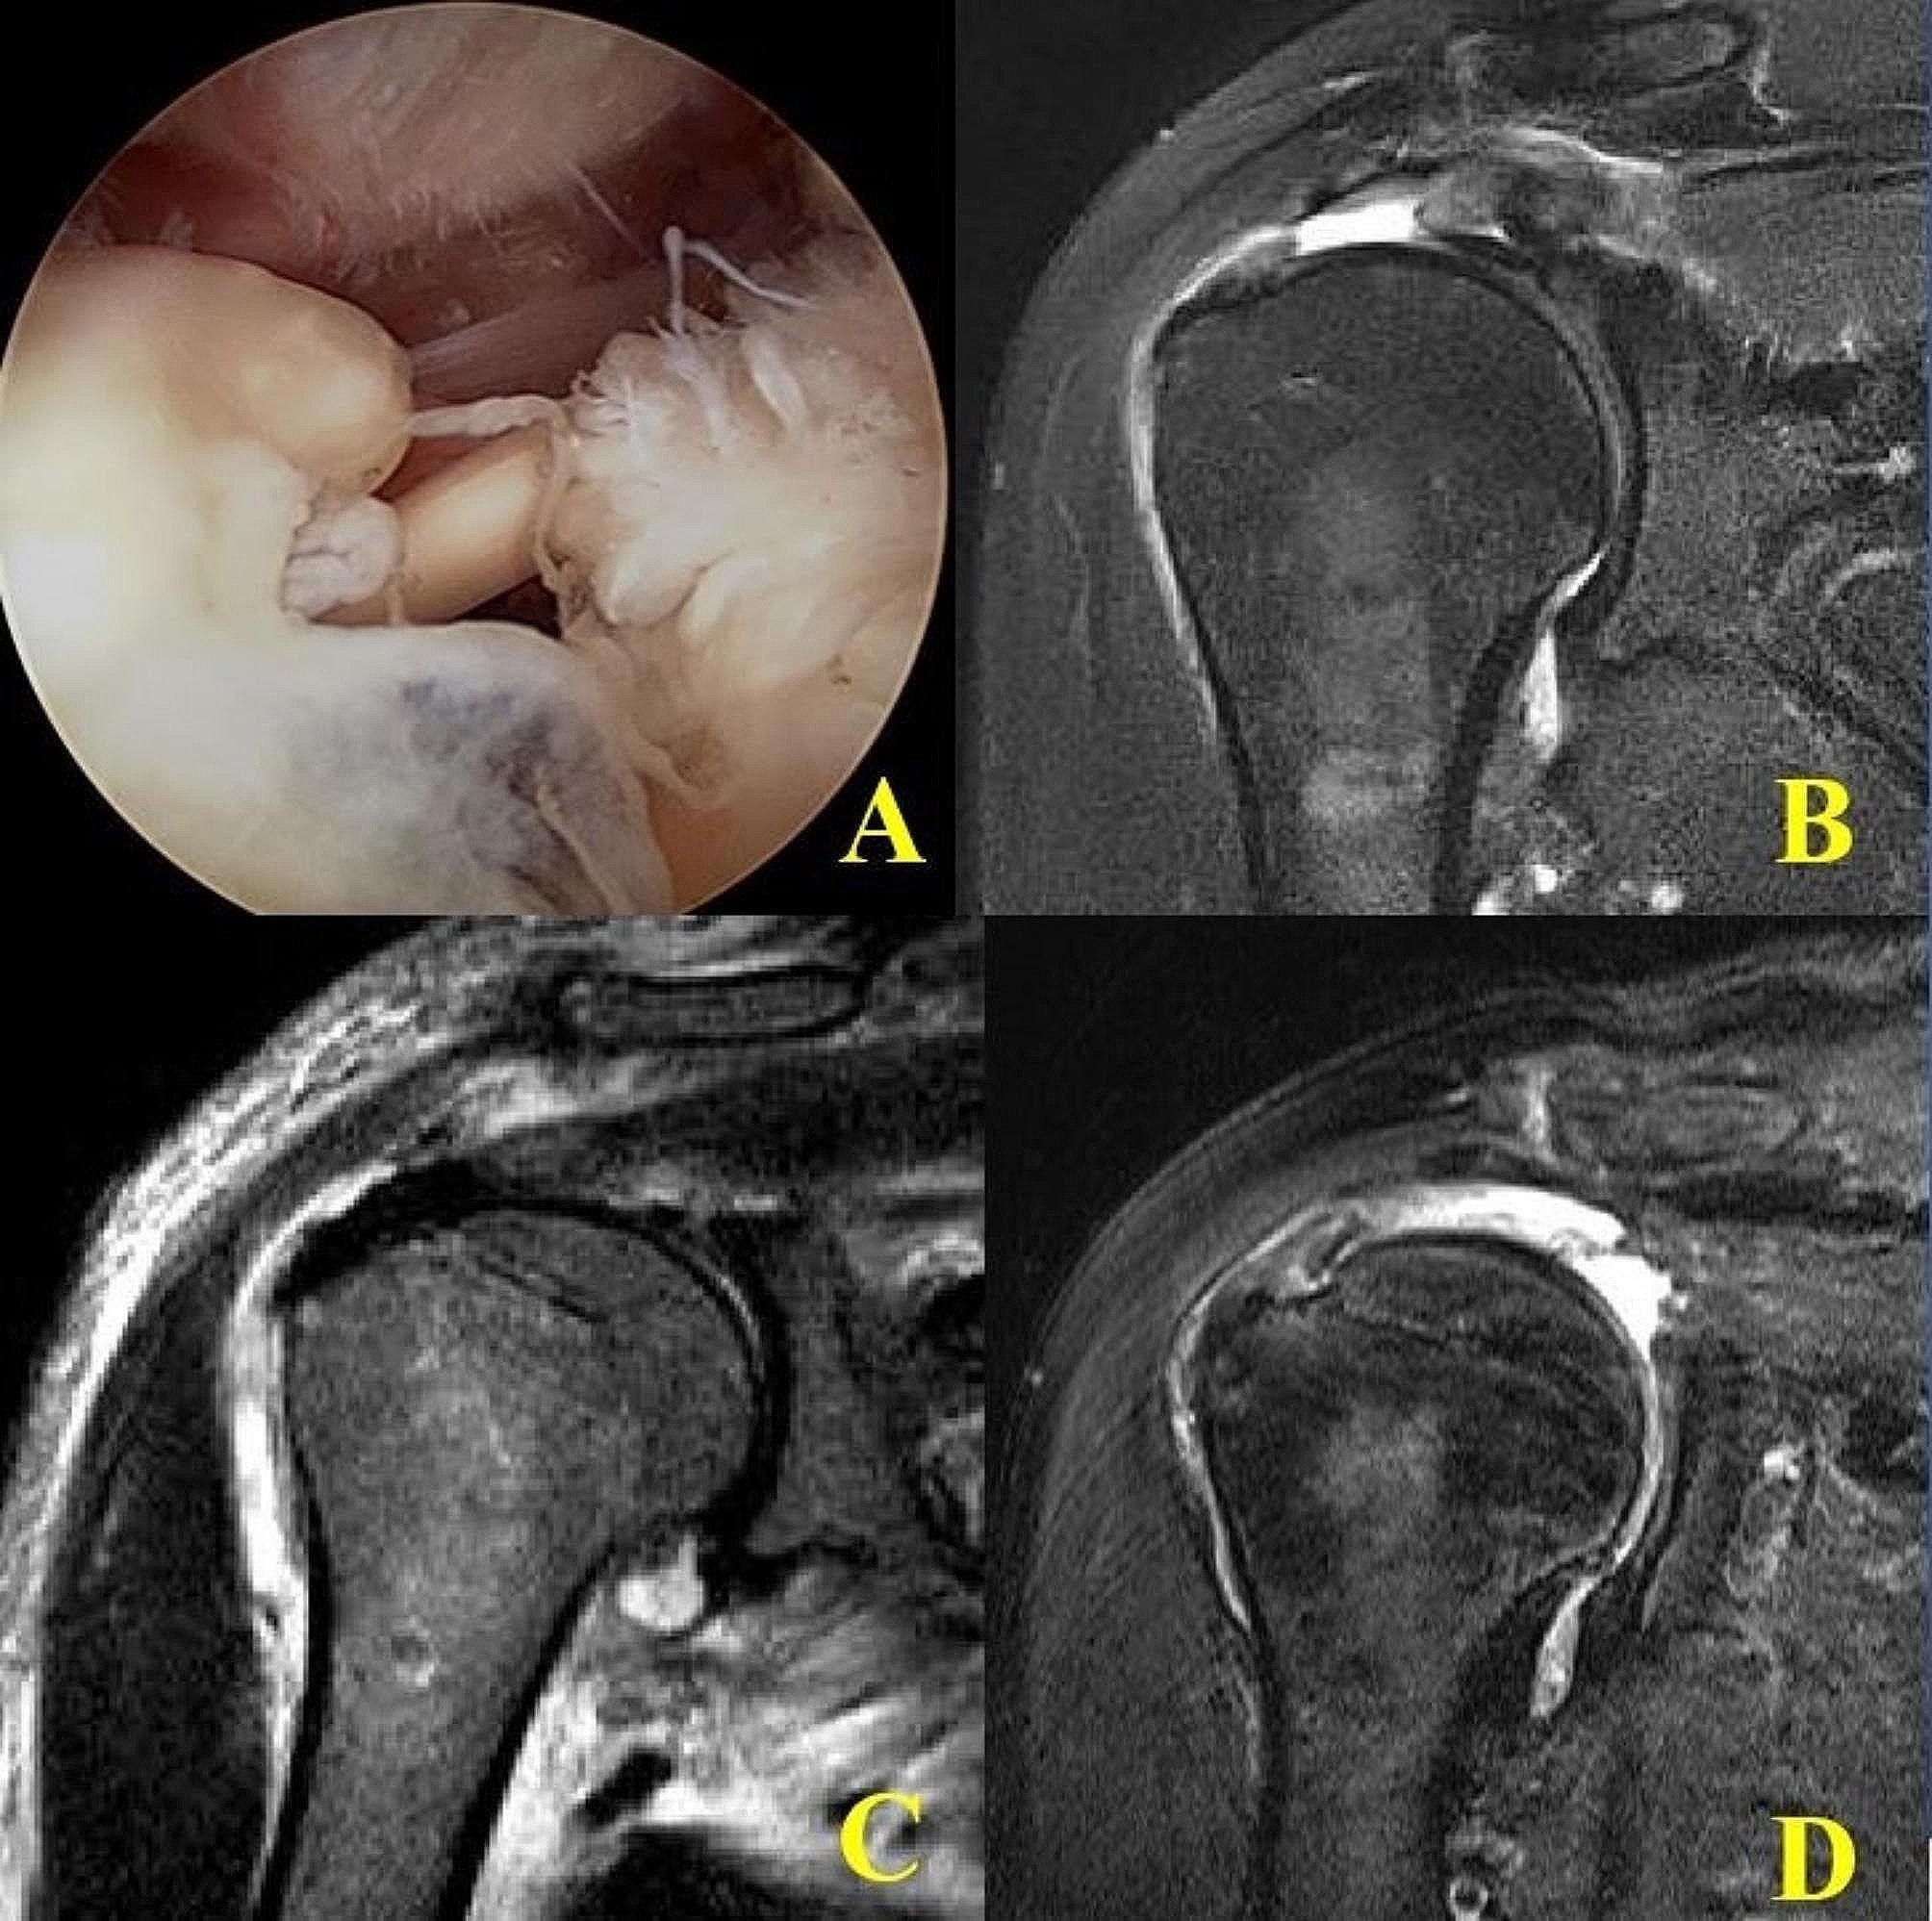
(A), (B) Arthroscopy and preoperative MRI showed that the cuff remnant on the footprint has a similar width to the original GT footprint and medially retracted tendon. (C) Immediate postoperative MRI showed good preservation of the remnant cuff on the greater tuberosity in the suture bridge rotator cuff repair. (D) 1-year of follow-up MRI showed large sized rotator cuff re-tear with remnant cuff (type 2 re-tear pattern)
